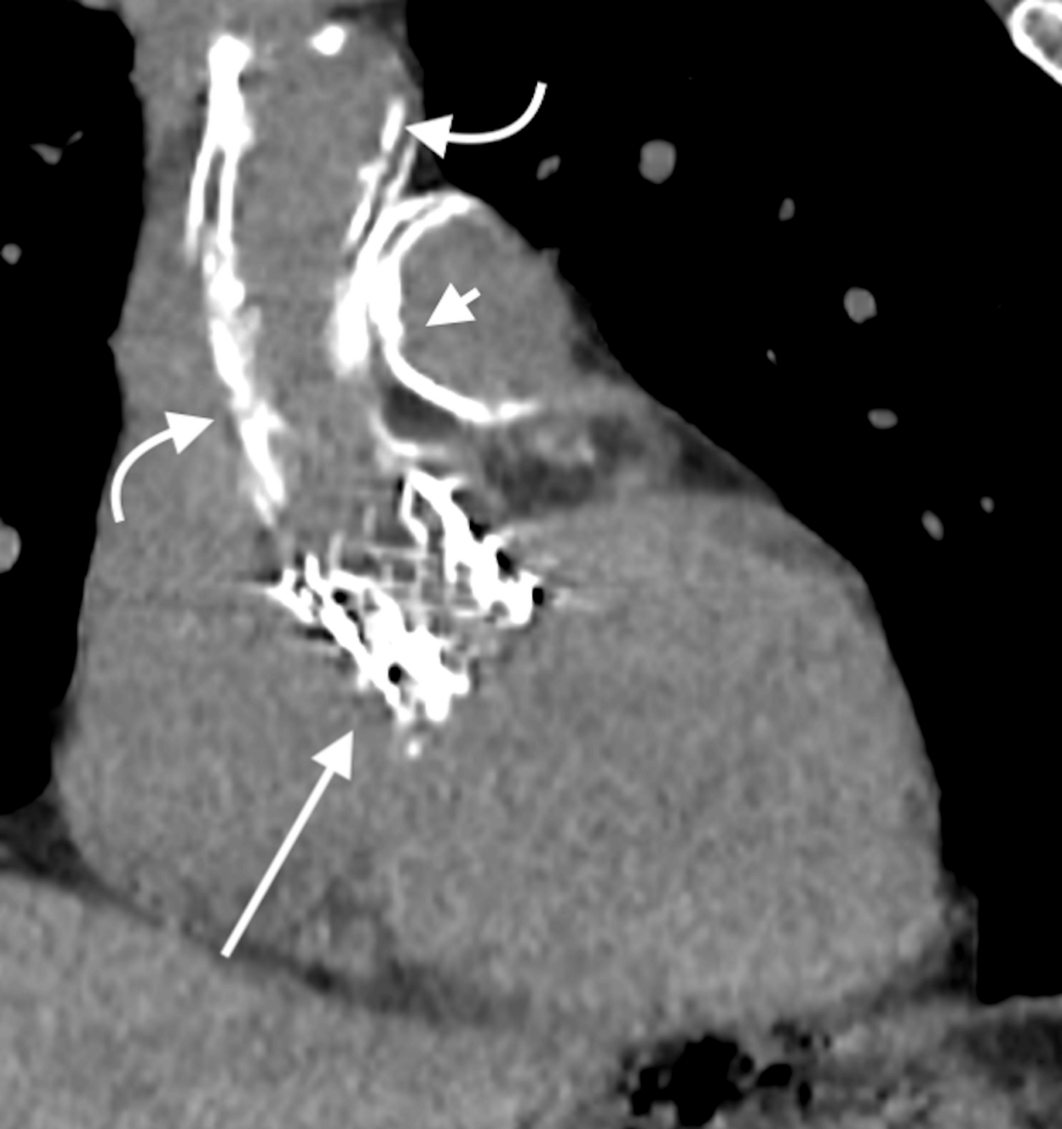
Coronal non-contrast CT in soft tissue window through the mediastinum. Shows severe atherosclerotic calcifications of the ascending aorta (curved arrows), a metallic mesh-like stent (long arrow) consistent with the patient’s history of TAVR, and severe calcifications of the pulmonary infundibulum and pulmonary valve (short arrow). TAVR: transcatheter aortic valve replacement
